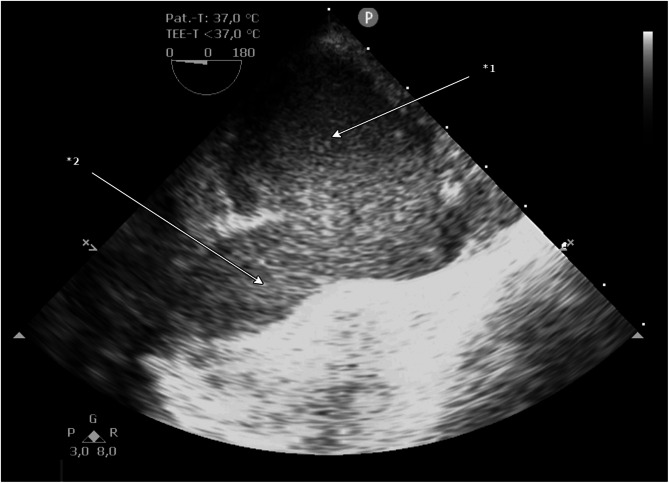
Transesophageal echocardiography of ruptured aorta with the pseudoaneurysm, long axis, *1 pseudoaneurysm; *2 aortic arch
